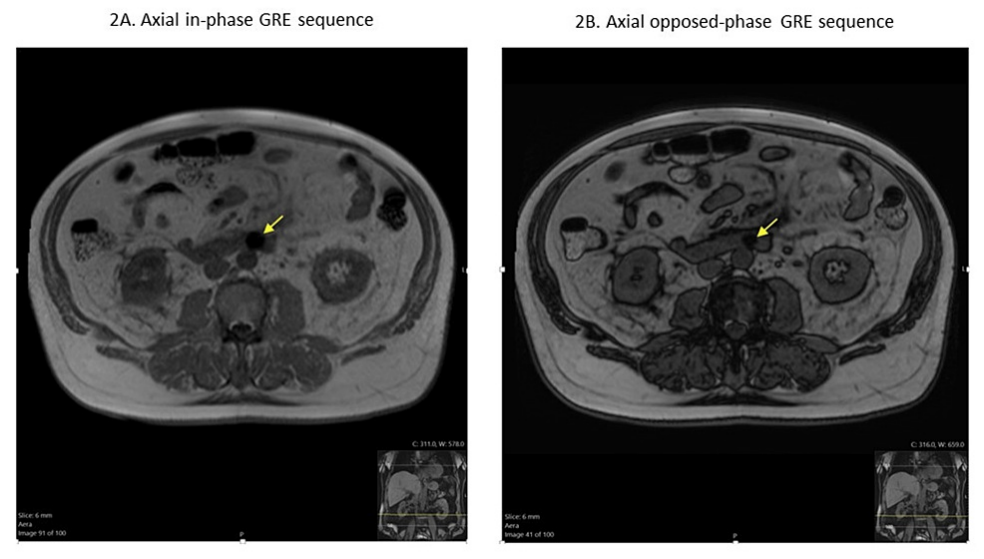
MRI of the abdomen and pelvis. Both sequences show signal loss (aka susceptibility artifact). More signal loss on in-phase (2A) compared to opposed phase (2B, known as blooming artifact) is indicative of paramagnetic material (weakly magnetic metals).
